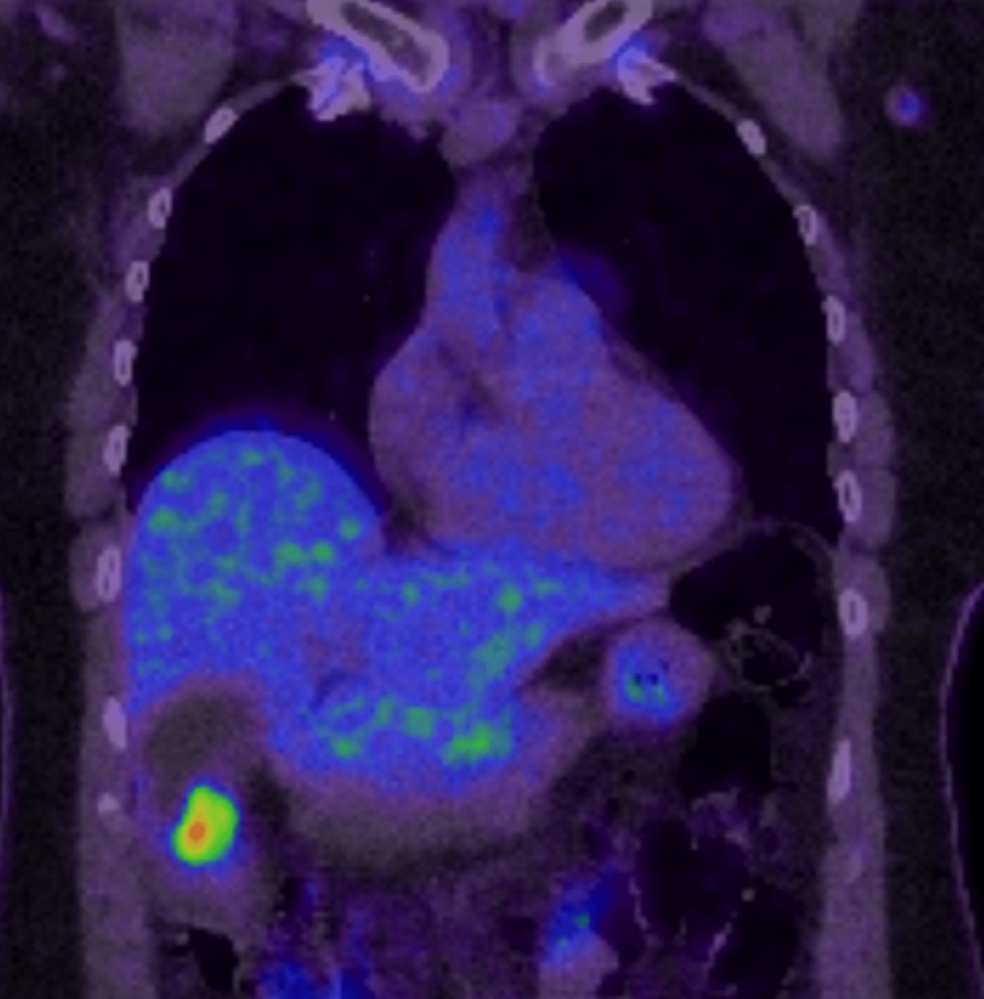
Moderate to intense fluorodeoxyglucose (FDG) avidity in the gallbladder body towards the fundus
